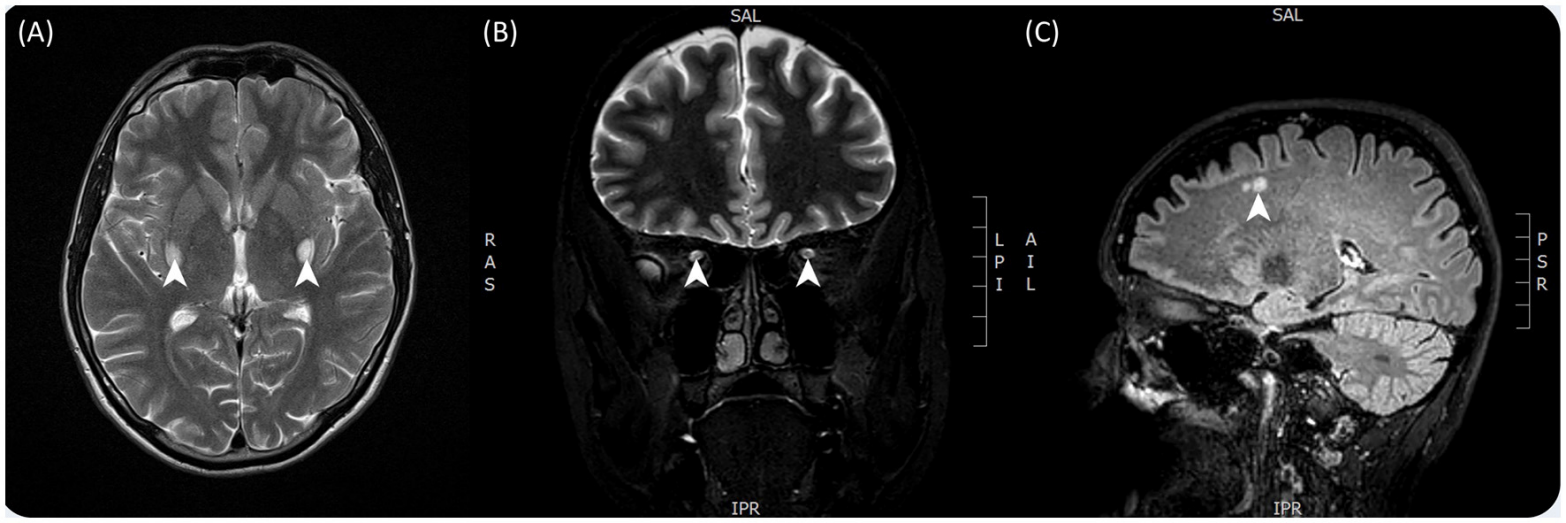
T2-weighted contrast-enhanced brain MRI images taken from both patients. (A) Taken from patient 1 and showing bilateral enhancements of the posterior basal ganglia in particular the putamen. (B) Taken from patient 2 and showing hyperintensity of the optic tracts bilaterally. (C) Taken from patient 2 and showing hyperintense lesions in the subcortical layer of the cerebrum.
